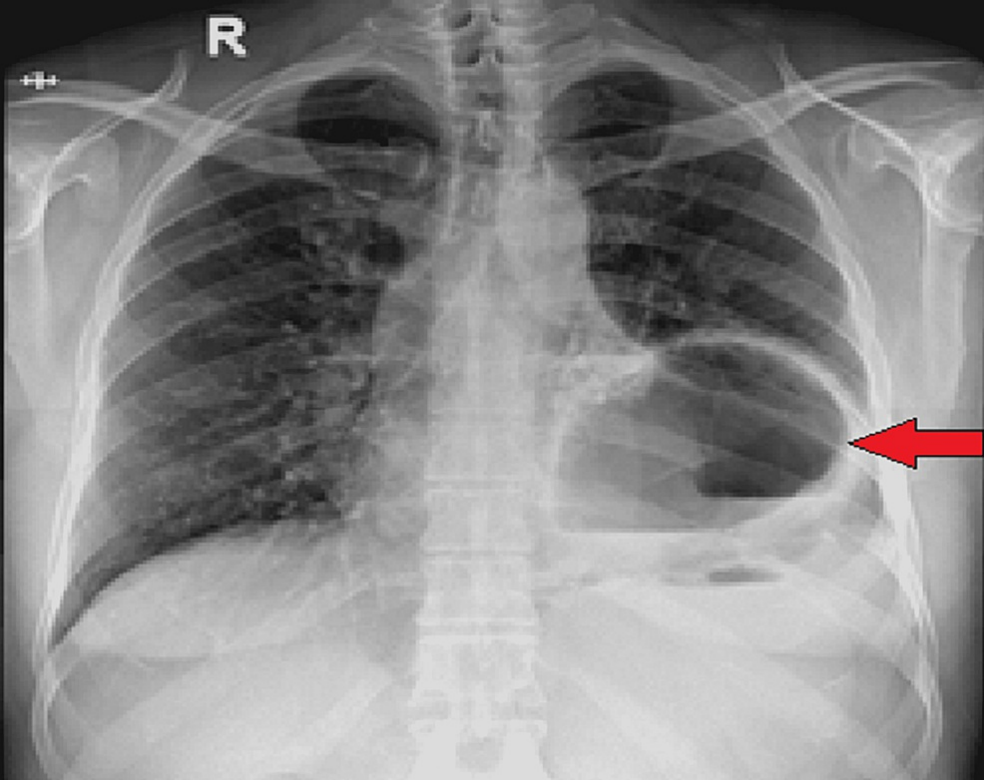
X-ray chest AP view showing a cystic area in left hemithorax demonstrating air-fluid level (red arrow) AP: anteroposterior
